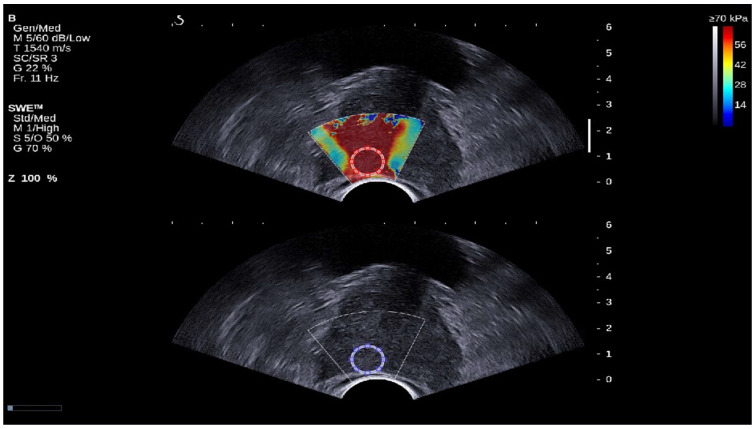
SWE images with b-mode ultrasound image of prostate cancer lesion. At the top (Red circle), the ROI is selected from the SWE image, and it is duplicated automatically in the B-mode ultrasound image (Blue circle). The red color map in the SWE image correspond to areas of higher stiffness, whereas the blue color map indicate areas of lower stiffness.
